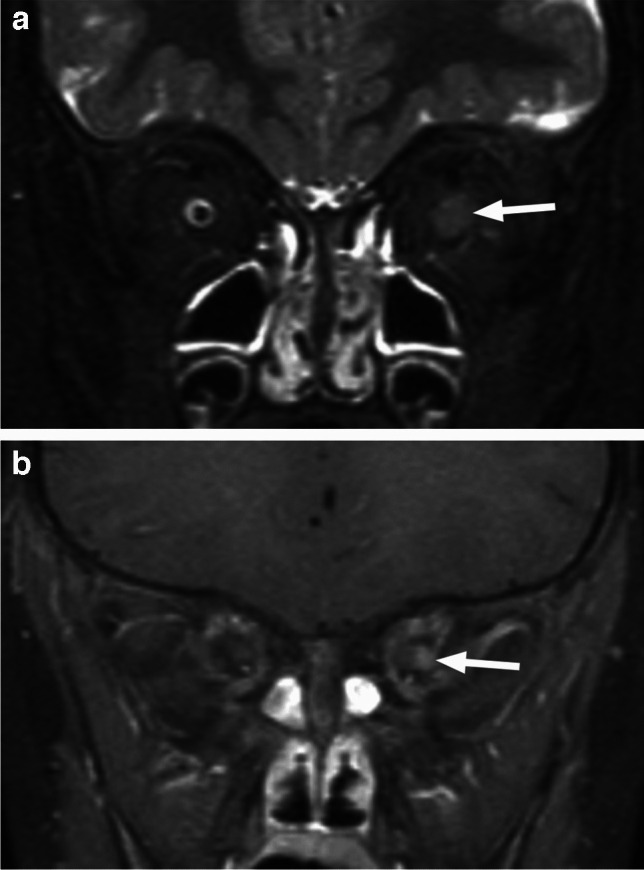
Coronal STIR image (a) shows thickening and increased signal intensity of the retrobulbar left optic nerve (arrow). There is also enhancement of the intraorbital nerve (arrow) on a more posterior coronal postcontrast T1-weighted image with fat saturation (b). The findings are consistent with PLONI in this patient with retinoblastoma of the left eye
